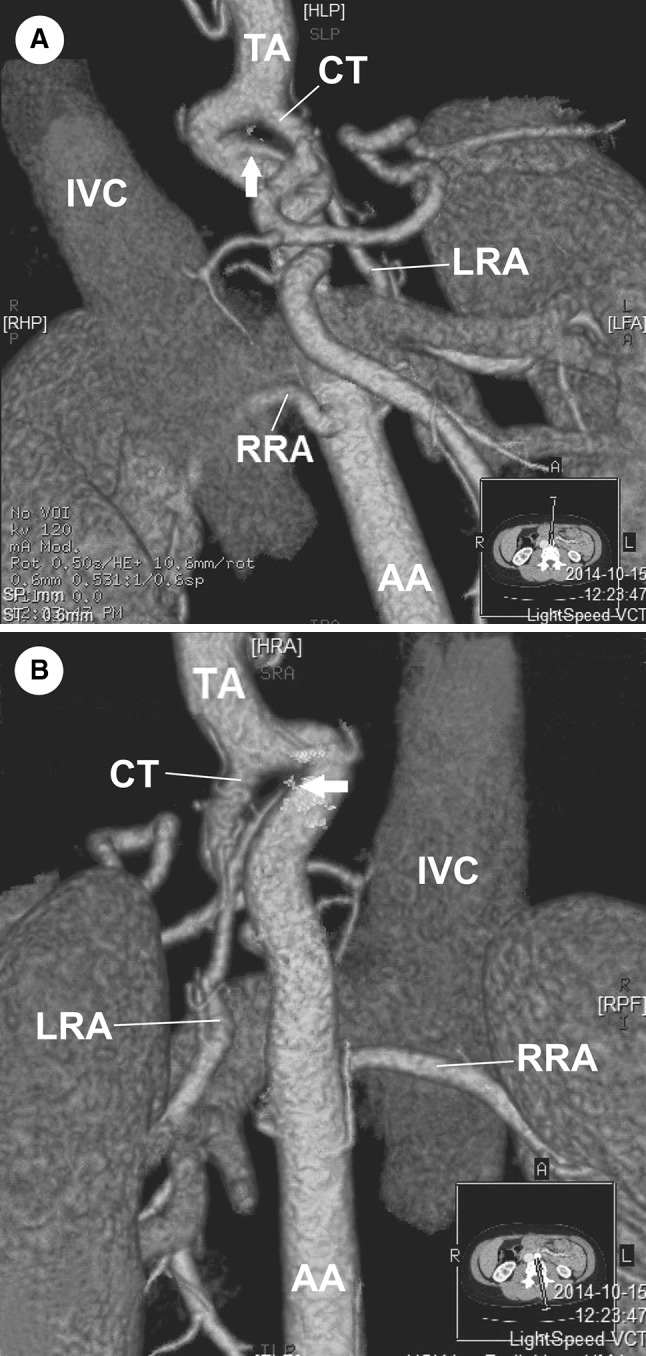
Abdominal computed tomography angiography, volume rendering. a Anterior view, b posterior view. *Arrow* origin of left renal artery, *AA* abdominal aorta, *CT* celiac trunk, *IVC* inferior vena cava, *LRA* left renal artery, *RRA* right renal artery, *TA* thoracic aorta
